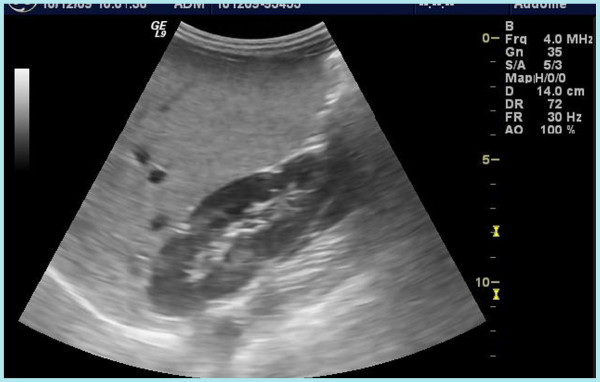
Abdominal ultrasound of CS with evidence of bright liver suggestive of severe hepatic steatosis.
